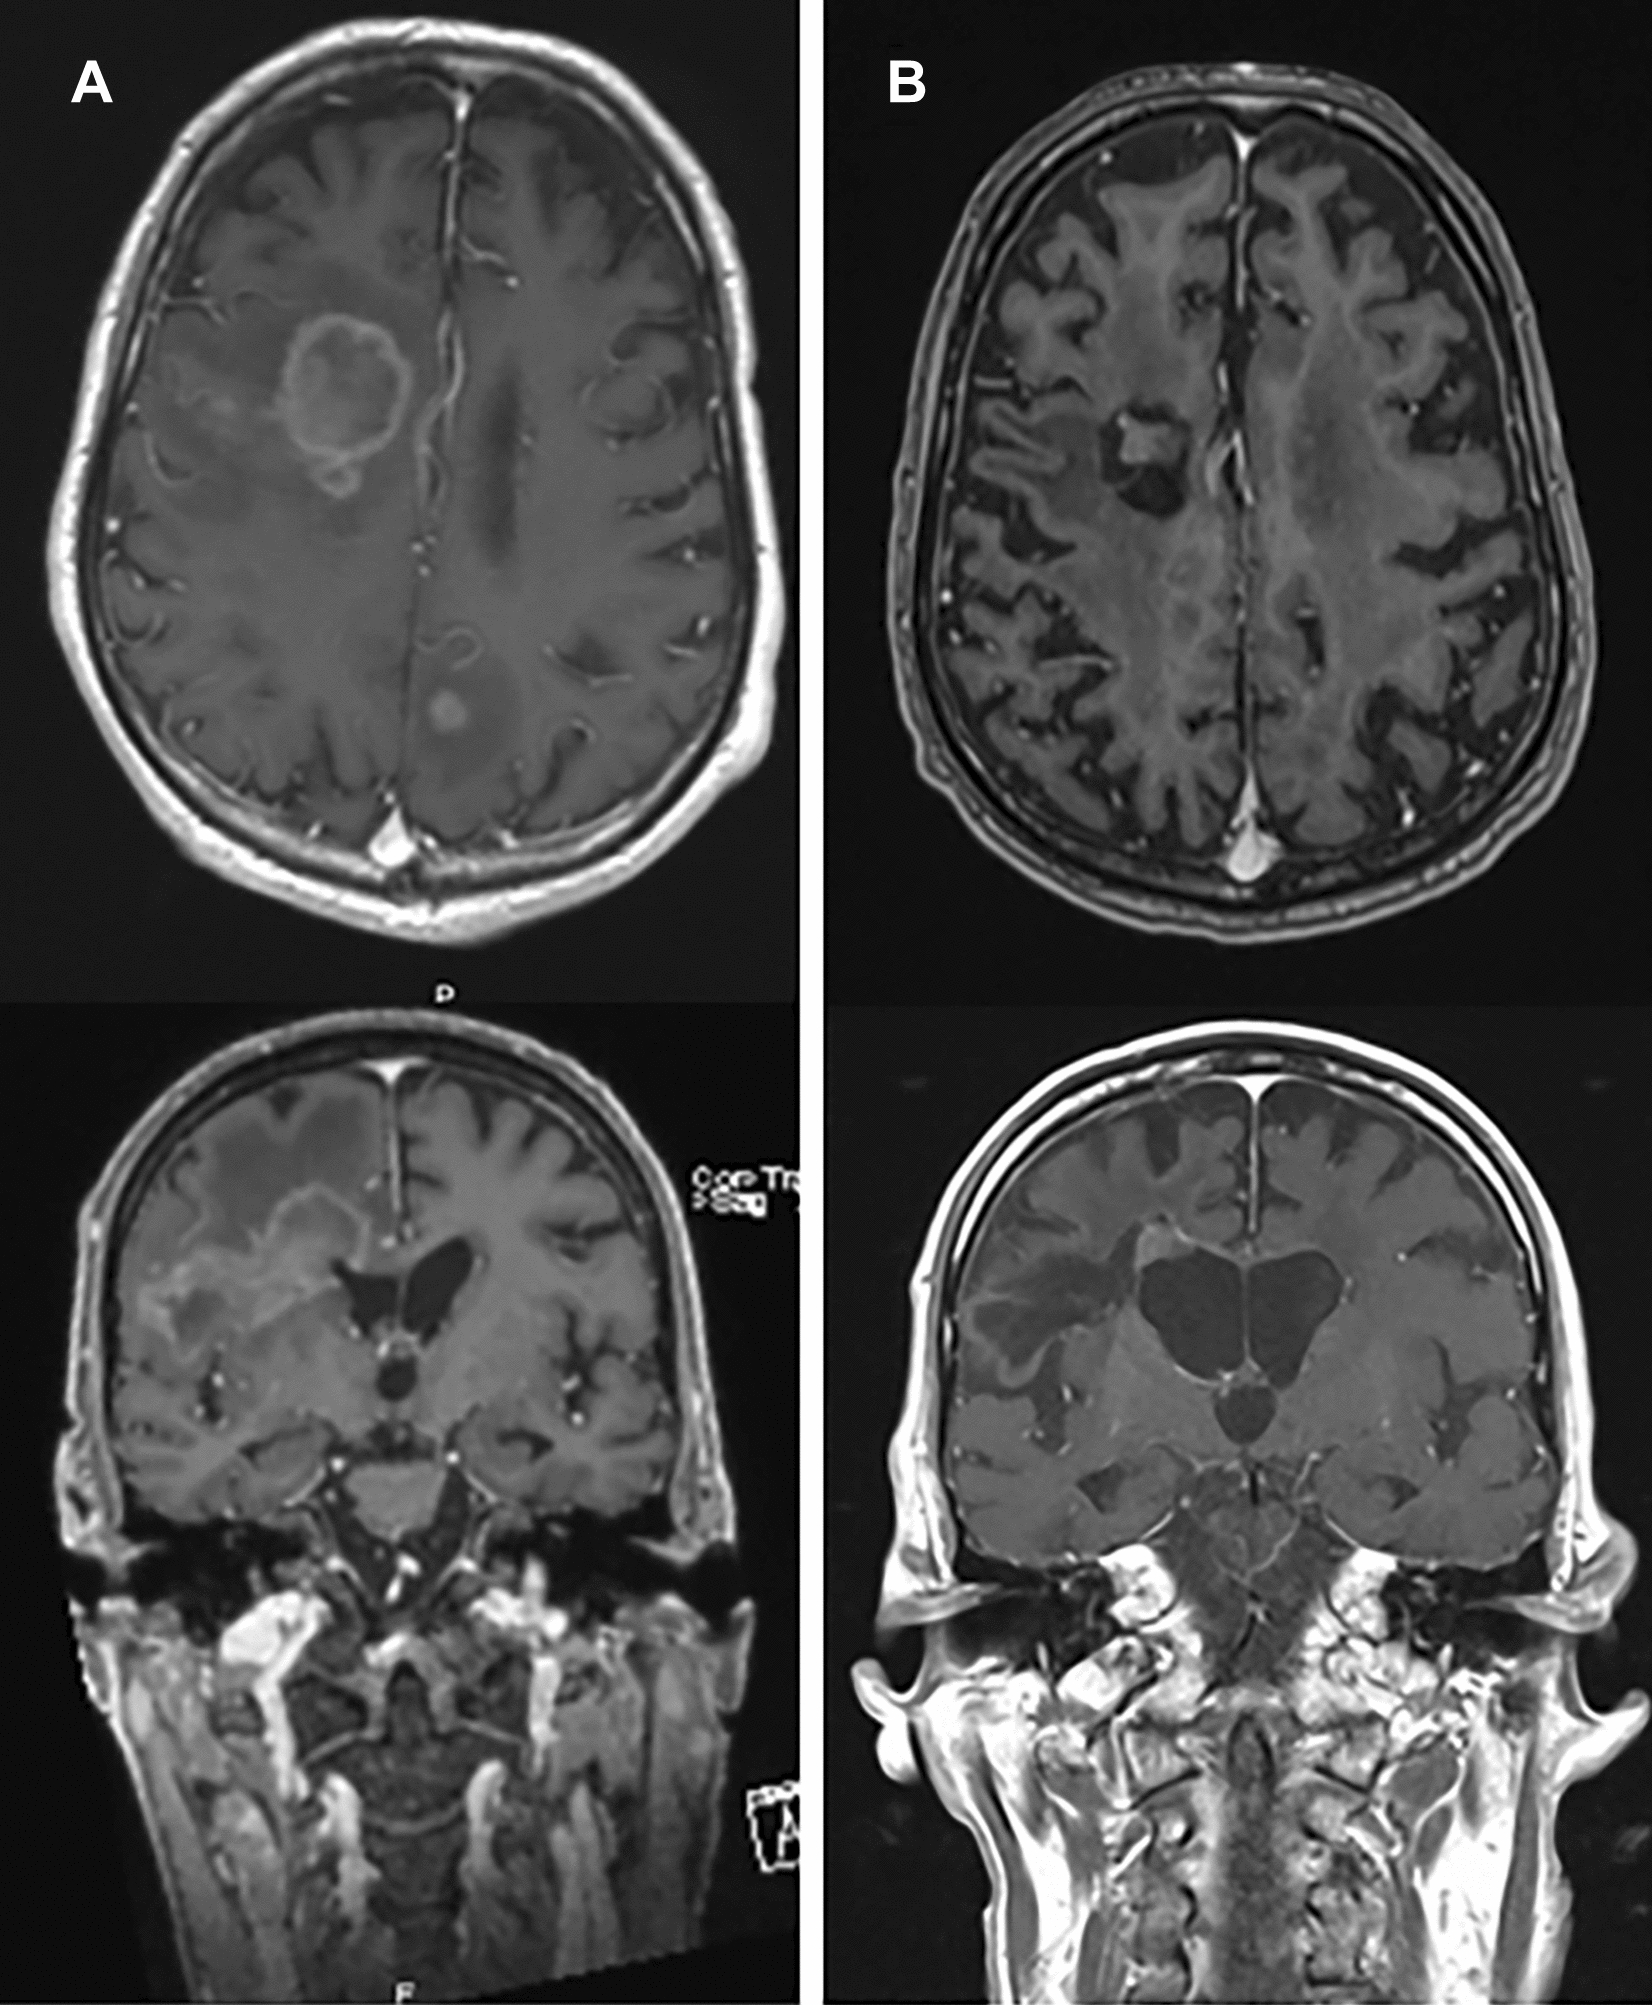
A T1-weighted with Gadolinium axial and coronal magnetic resonance image of brain showing a ring enhanced brain lesion involving the right frontal lobe. B T1-weighted with Gadolinium axial and coronal magnetic resonance image showing a complete remission of the lesion
